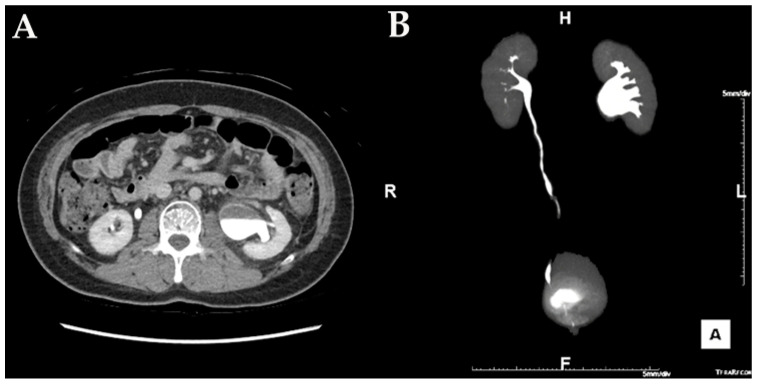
In the abdominal CT, hydronephrosis with a UPJ obstruction on left urinary tract is noted. (A) axial image of the left UPJO, (B) reconstructed coronal image of the left UPJO.
